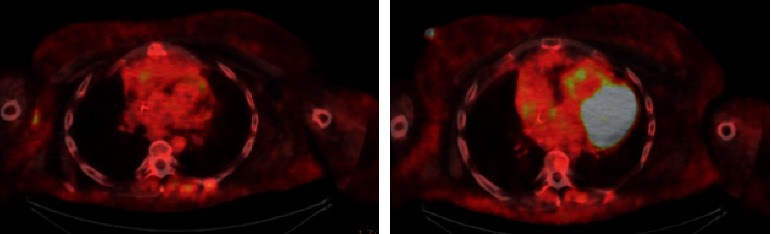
PET/CT after 2 rounds of DRD therapy showing significant improvement in hypermetabolic activity seen in previous imaging consistent with complete response (Deauville 2).
